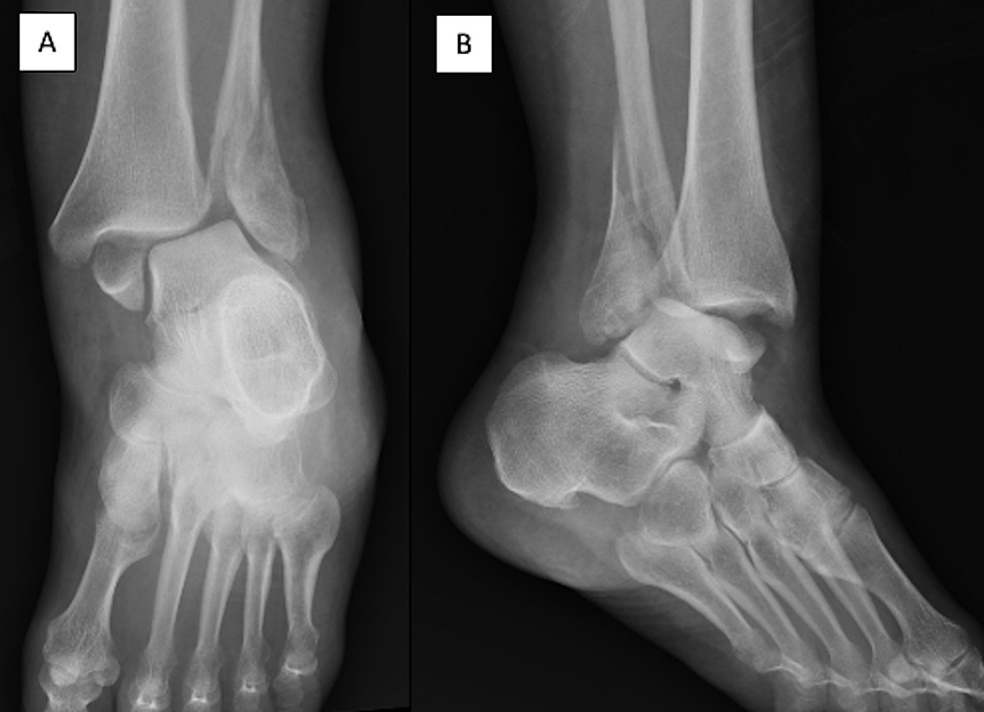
Index injury radiographs. AP (A) and lateral (B) radiographs of the right ankle demonstrating a bimalleolar ankle fracture-dislocation. AP = anteroposterior
